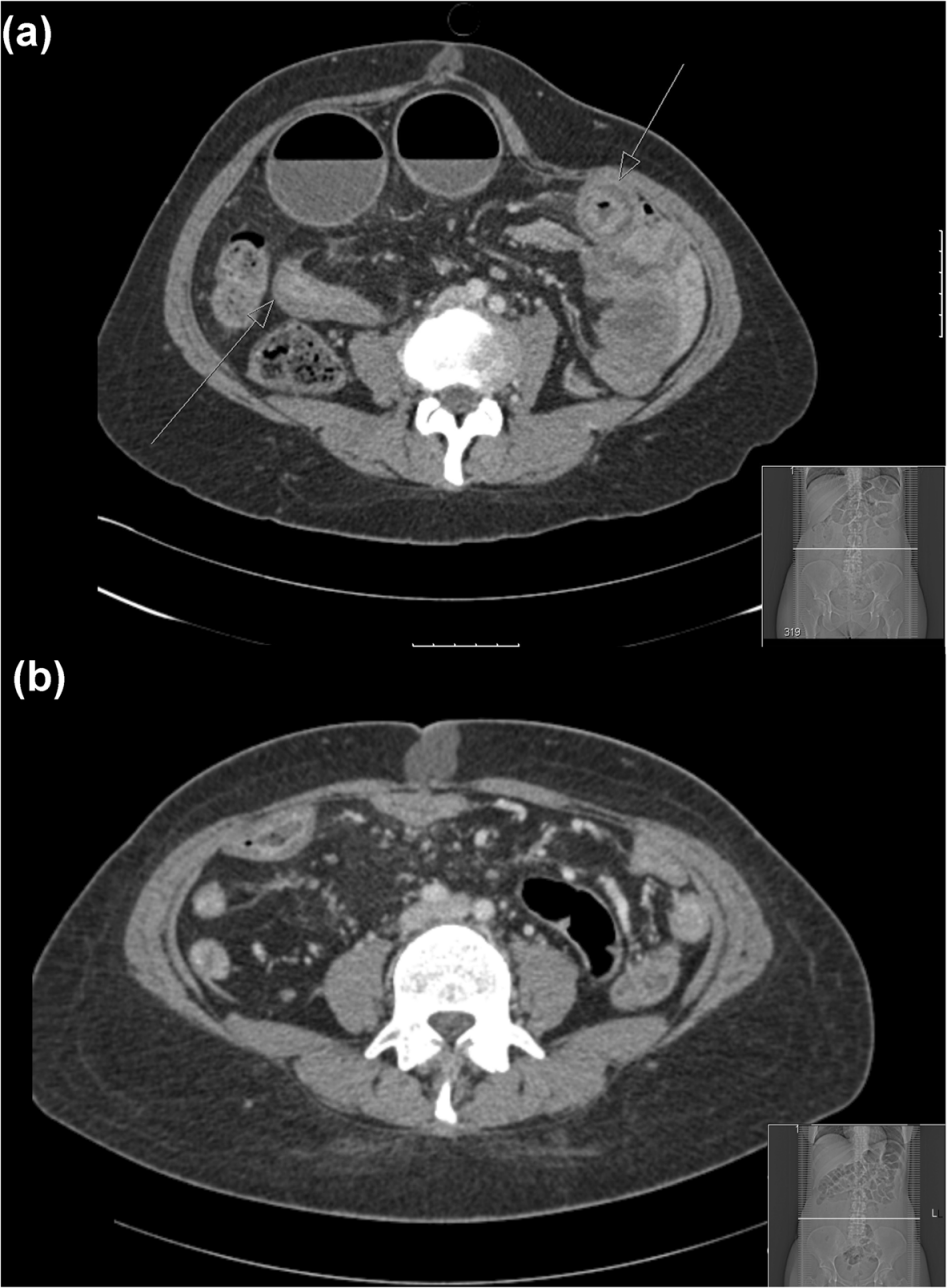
Initial computed tomography (CT) scan demonstrating (a) Crohn’s strictures; (b) Absence of mesenteric haematoma.
